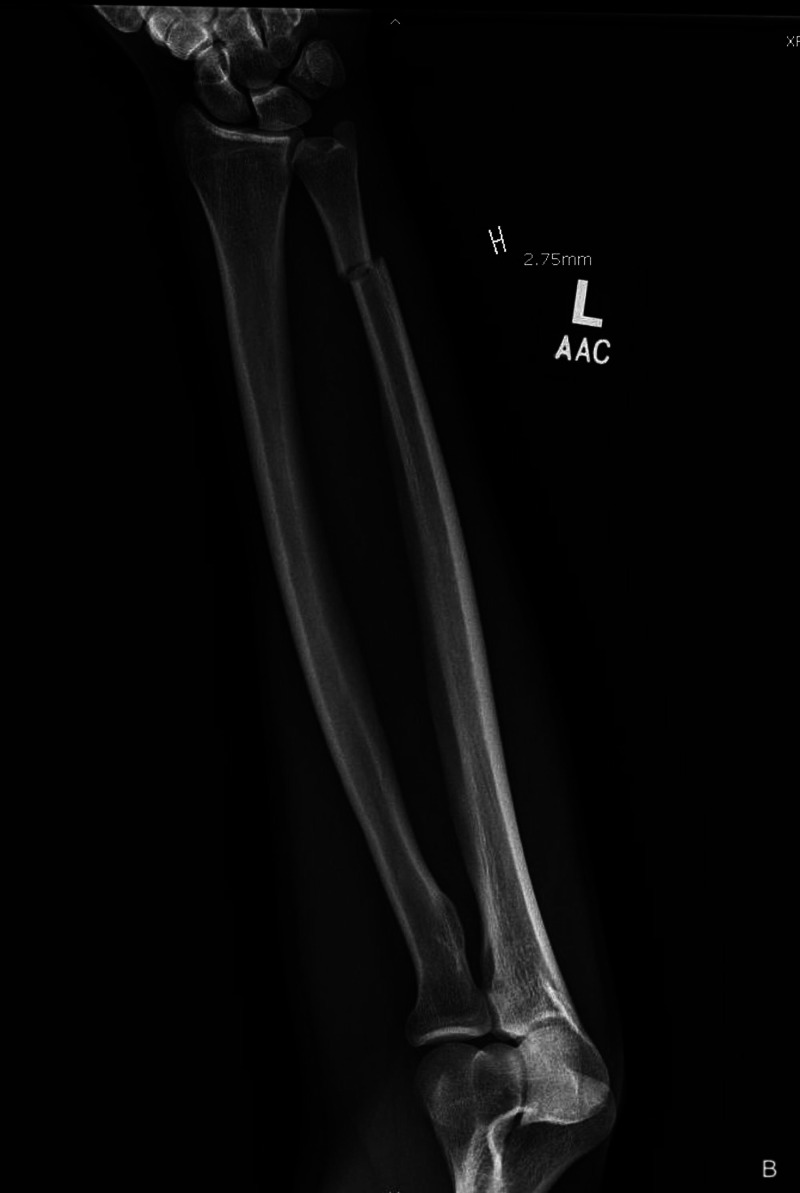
Anteroposterior injury film/radiograph of the left forearm demonstrating an isolated ulnar shaft fracture with minimal displacement and angulation.
